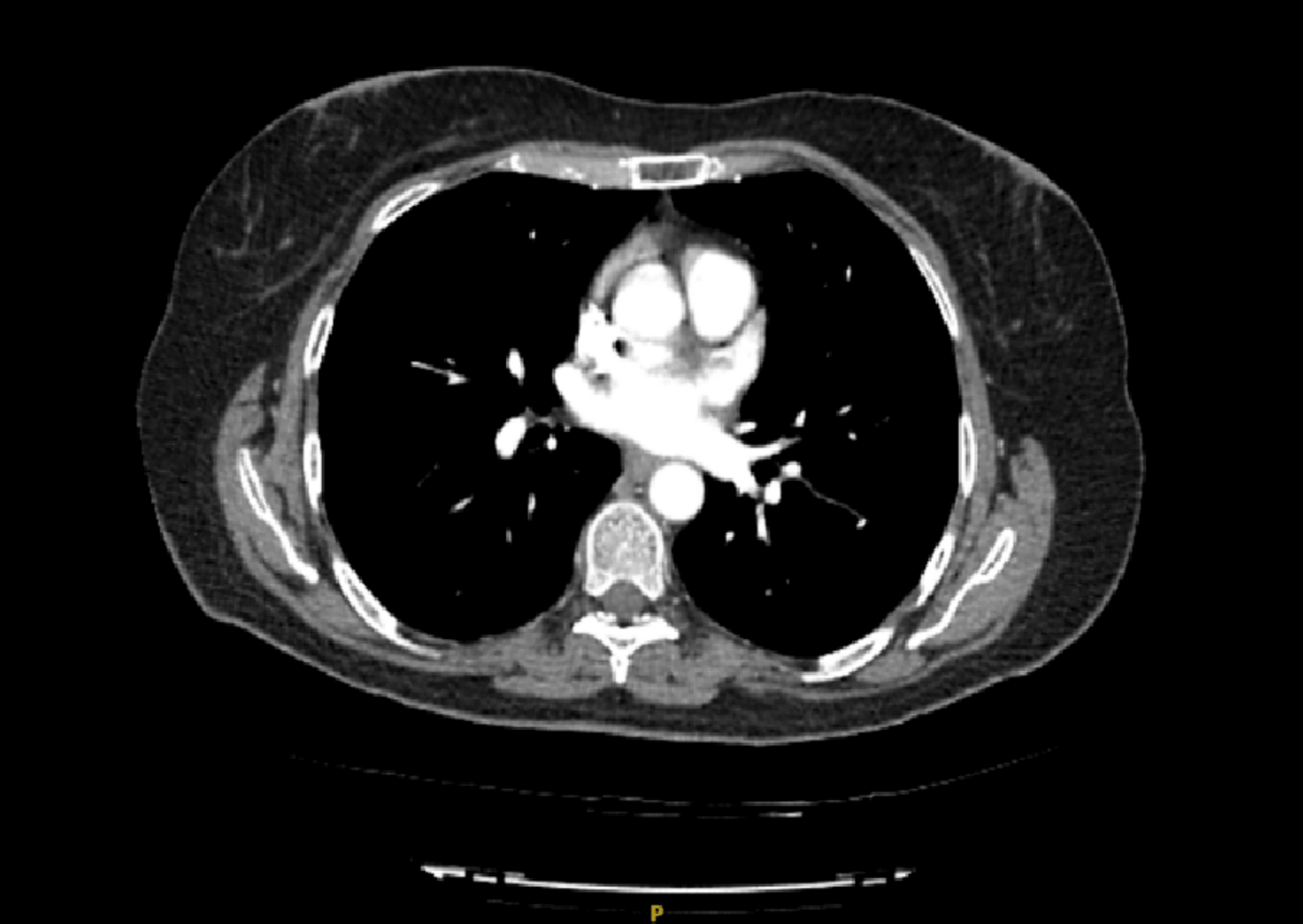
CTPA cross-sectional image The CTPA did not show any pulmonary emboli. CTPA: computed tomography pulmonary angiogram
